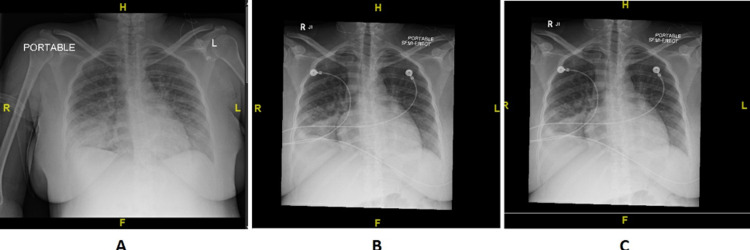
Chest X-ray showing improvement in vascular congestion.
